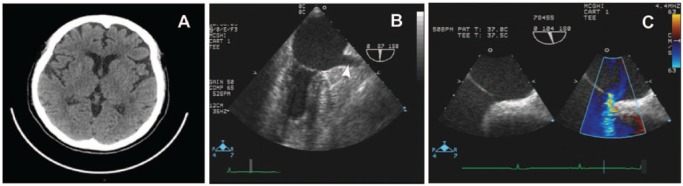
(A) CT head without contrast found no evidence of acute intracranial process such as thrombus, hemorrhage, mass effect, or hydrocephalus. (B, C) TEE images showed no evidence of thrombus in the left atrial appendage nor valvular vegetations. It showed a normal left ventricular function with ejection fraction of greater than 55% and secondum ASD (8.2 mm size with qp:qs ratio of 1.6:1).
